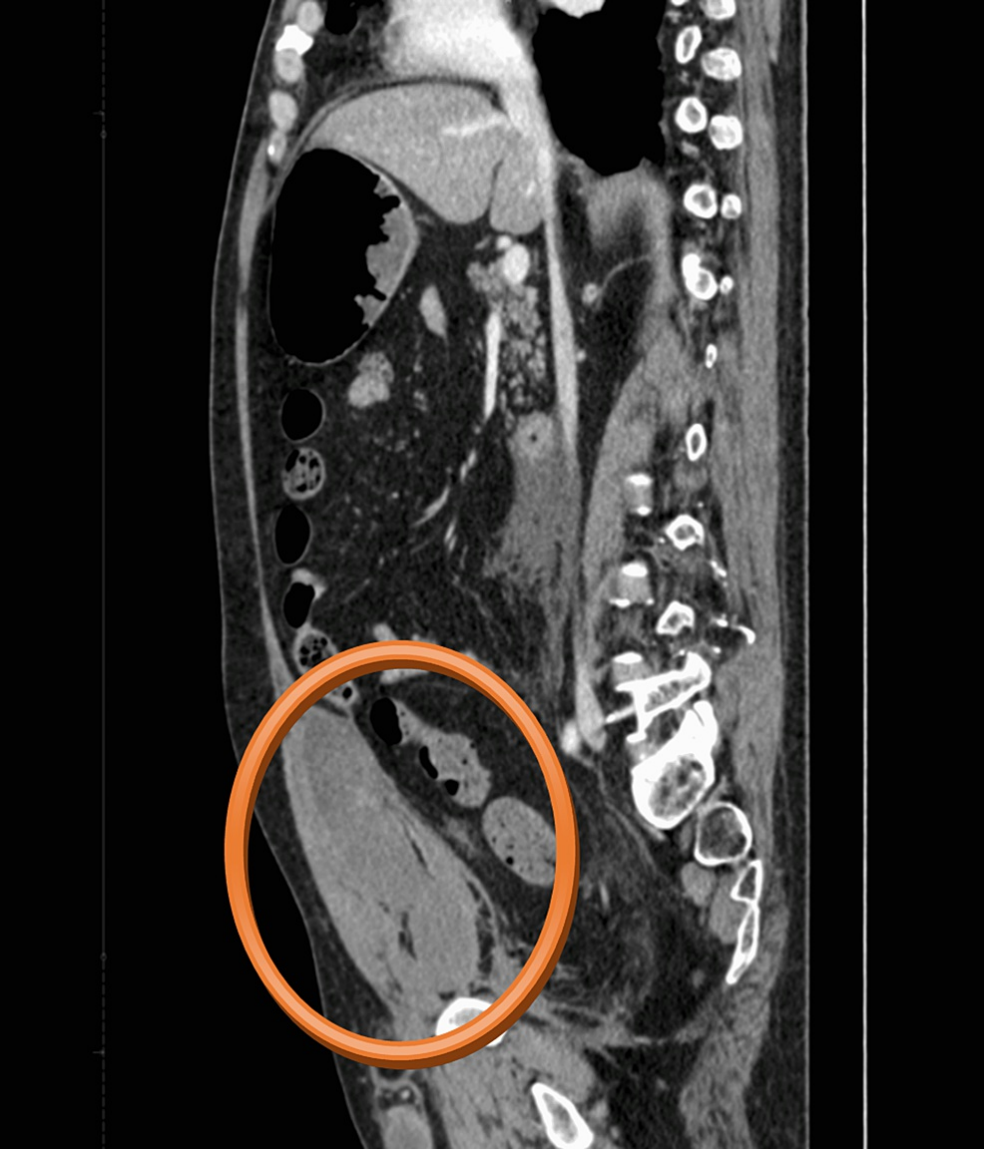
CT scan - sagittal plane Hematoma of size 130 x 80 x 50 mm in the right musculus rectus abdominis can be seen
